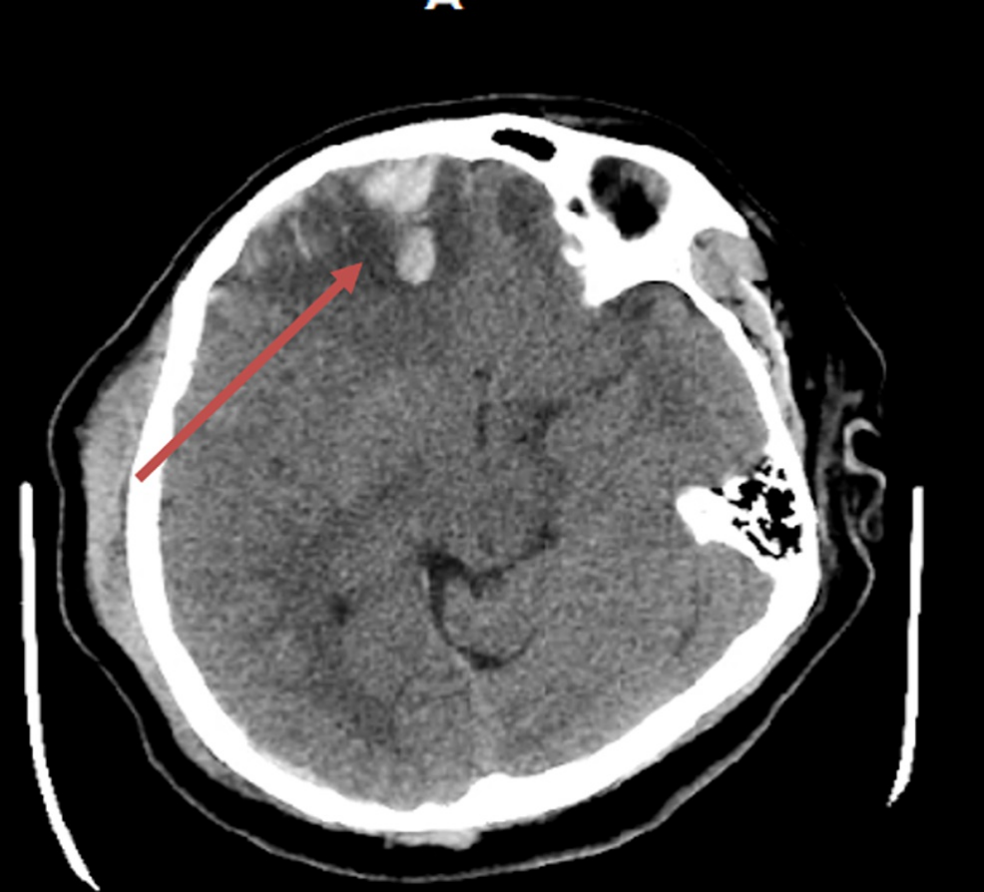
Brain CT at 48 hours showing the worsening progression of intracranial injuries (arrow)
